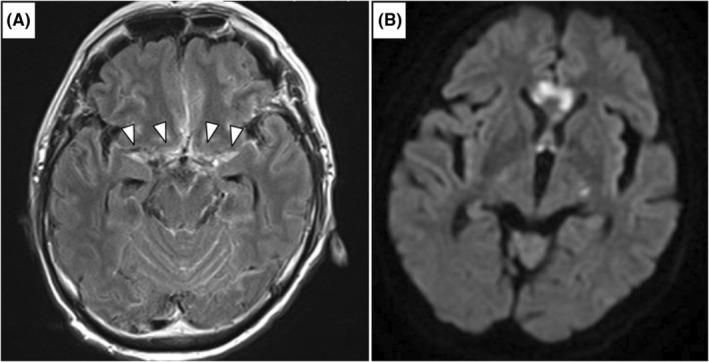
Head magnetic resonance imaging. The basal meningeal enhancement was observed (A; gadolinium‐enhanced fluid‐attenuated inversion recovery image: arrow heads), along with multiple infarctions mainly at the corpus callosum (B; diffusion‐weighted image). Tuberculoma, hydrocephalus, and vasculitis were not found.
